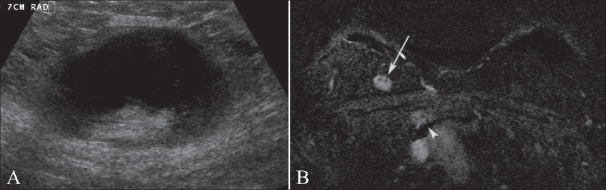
USG of an enlarged axillary lymph node (A) and fat-saturated axial DCE-MRI subtracted image (B). This was a 48-year-old woman who initially presented with a palpable lump in the axilla. Axillary metastasis (A) due to an unknown primary was confirmed. Breast MRI identified a suspicious lesion (arrow in B) in the upper inner quadrant of the right breast. A second-look USG and biopsy followed, which confirmed the lesion to be the site of a primary breast malignancy. Also note the internal mammary lymphadenopathy (arrowhead in B)
